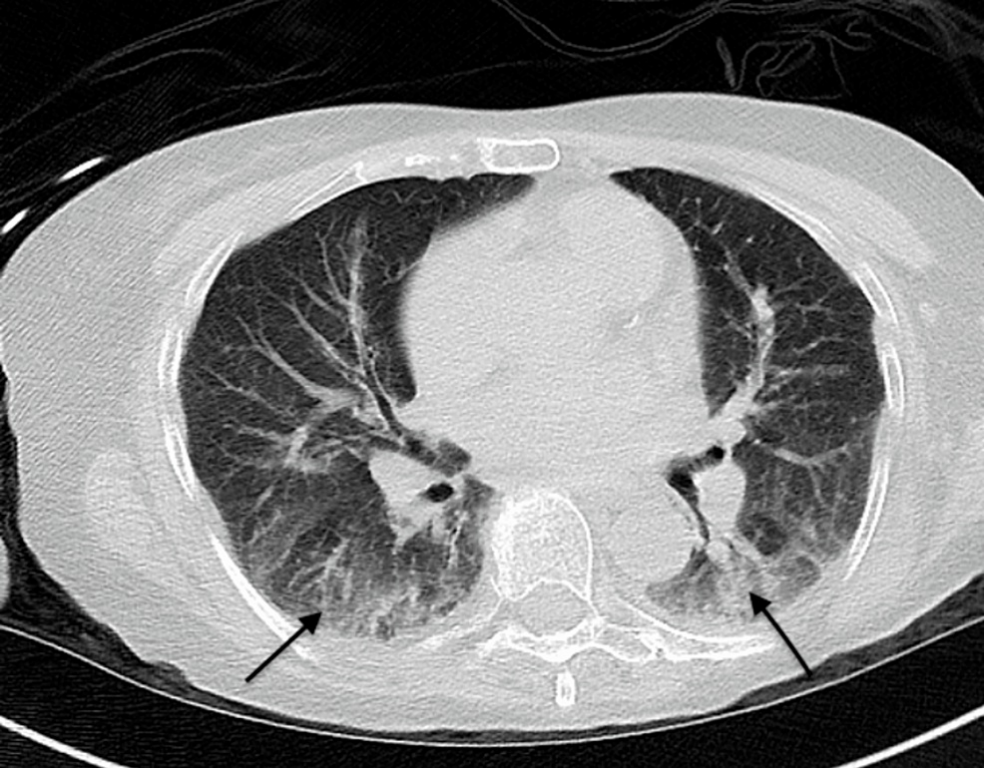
CT chest showing bilateral basal atelectasis
